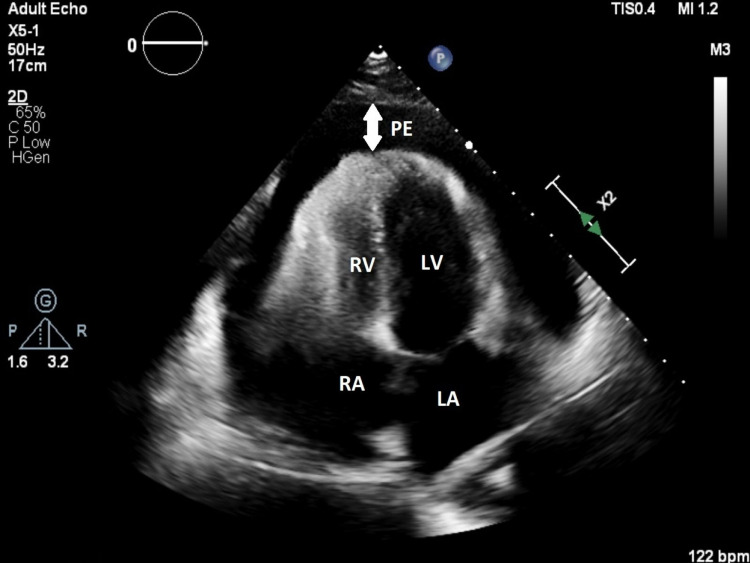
Transthoracic echocardiogram (apical view) revealing pericardial effusion LA - left atrium; RA - right atrium; LV - left ventricle; RV - right ventricle; PE - pericardial effusion
